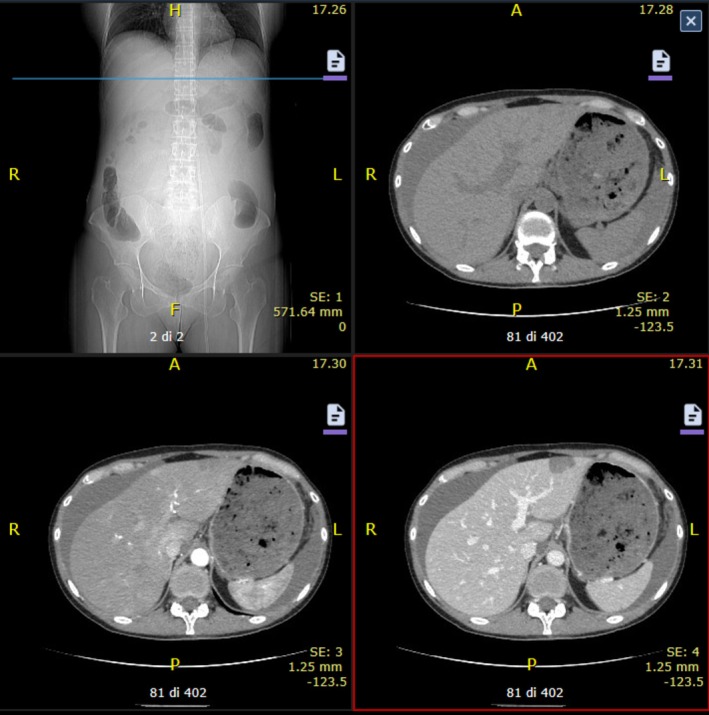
Abdominal computed tomography (CT) scan with and without contrast showing abundant abdominal free fluid, perihepatic (3 cm) and in the left hypochondrium (2 cm).
